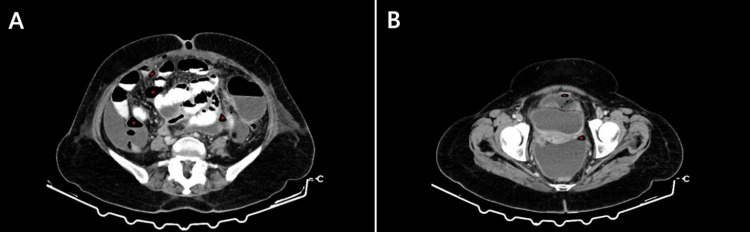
CT scan of the lower abdomen showing signs of peritonitis and encapsulated collections caused by perforation of the small intestine. Red asterisks: multiple encapsulated collections with air-fluid levels throughout the peritoneal cavity, located at (A): the paracolic regions bilaterally and (B): at the pouch of Douglas.
